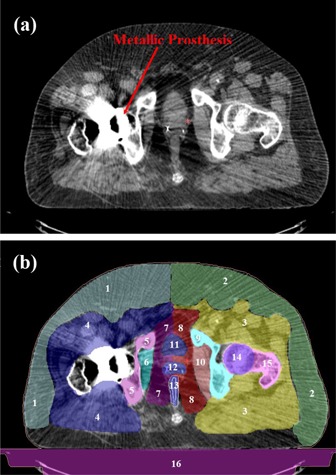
A transversal view of the computed tomography (CT) slice (case #4) showing (a) streaking artifacts produced by a metallic hip prosthesis, and (b) contoured artifacts and structures, which are numbered from 1 to 16. Details of each structure (1‐16) and their relative stopping power values are provided in Table 1.
